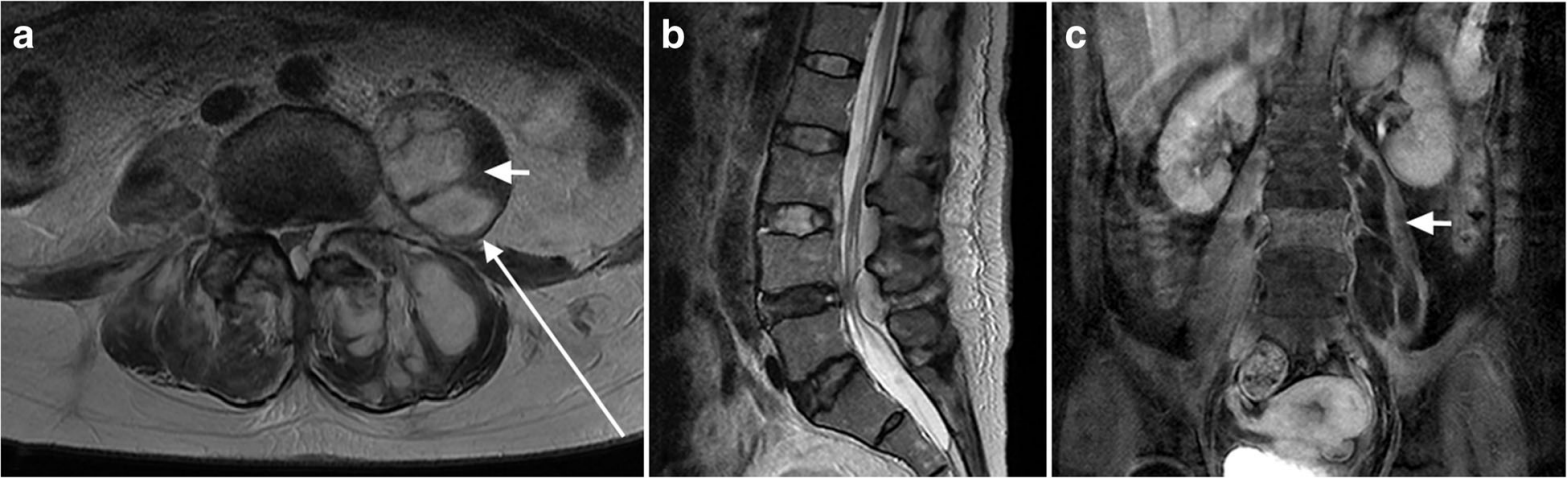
Magnetic resonance images of the lumbar vertebra before surgery. T2-weighted magnetic resonance imaging a L4–5 level, b sagittal view, and c coronal gadolinium-enhanced magnetic resonance imaging showing psoas, epidural, and paravertebral abscesses. The *short white arrow* shows the location of psoas abscess and the *long white arrow* shows the pathway of percutaneous endoscopy to the psoas abscess. The third vertebra is enhanced by gadolinium and diagnosed as pyogenic spondylitis (c)
